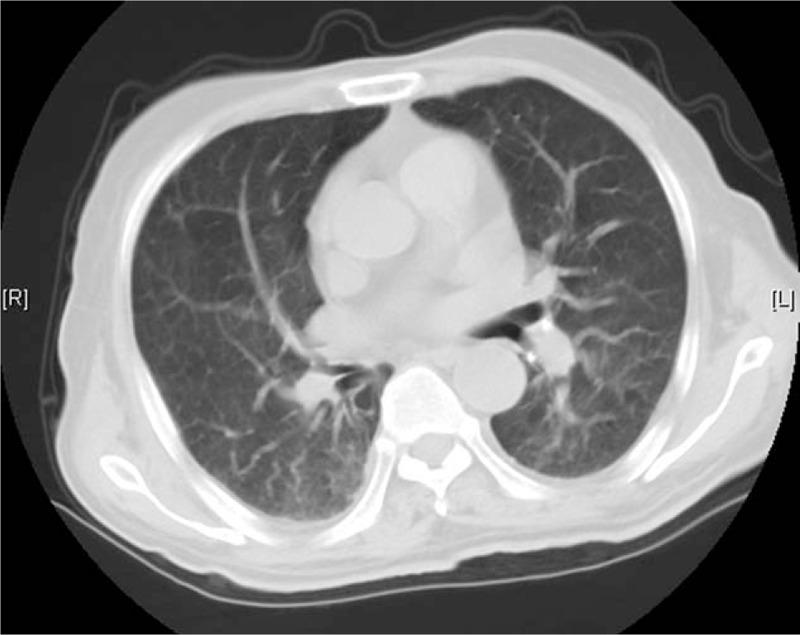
A chest computed tomography showing interstitial pneumonia.
